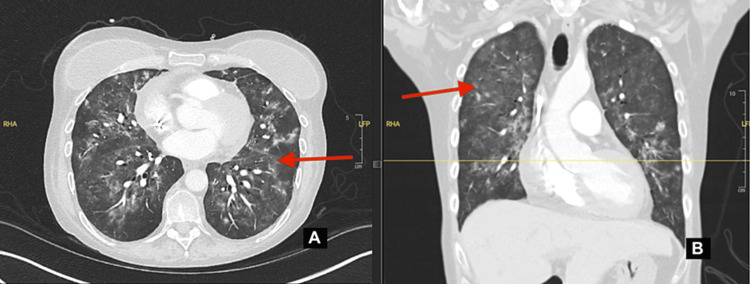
Axial (A) and coronal (B) CT pulmonary angiographies demonstrating extensive bilateral ground-glass opacities and septal thickening.
